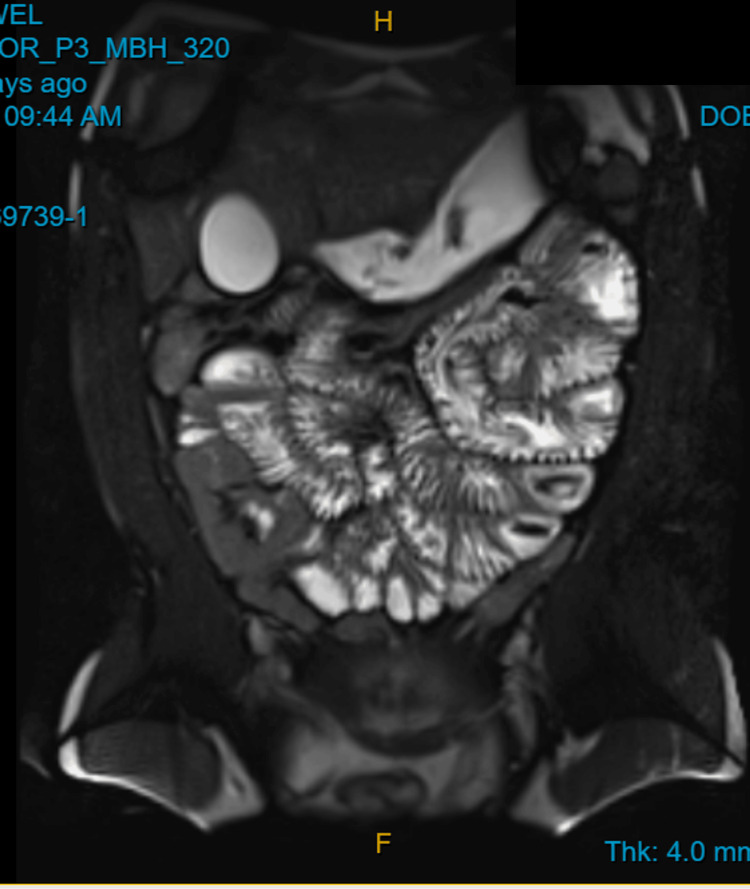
MRI showing no abnormalities.
